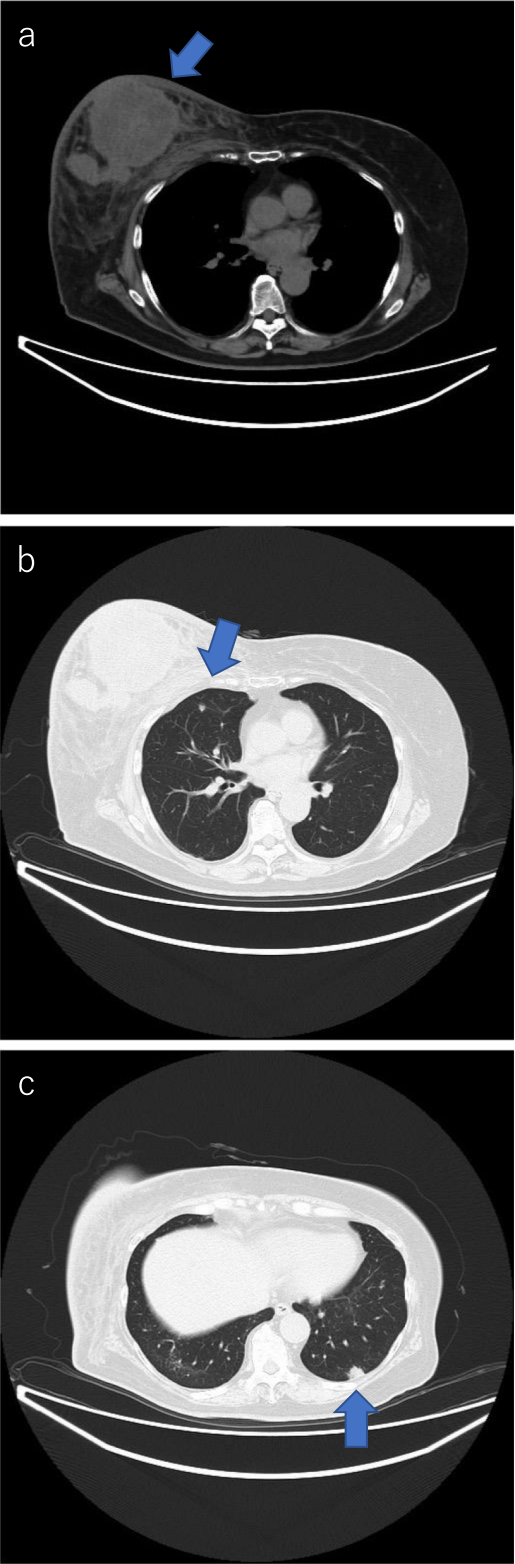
CT before chemotherapy shows left breast tumor, normal aorta (a), and multiple lung metastases (b, c)
